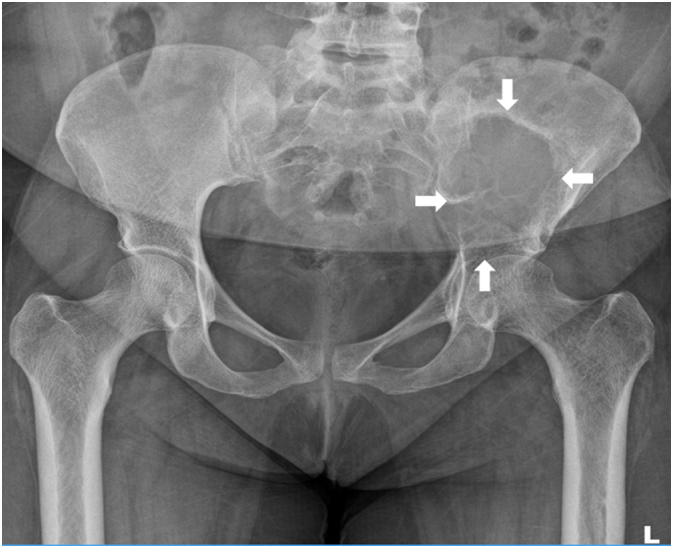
X-ray of the pelvis demonstrated a large lytic bubbly lesion (solid white arrows) within the left iliac wing extending to involve the left acetabulum but sparing the left femur demonstrating a lobulated sclerotic superior border and ill defined inferior, medial and lateral borders suggestive of a bony metastasis in the context of a malignant thyroid mass.
